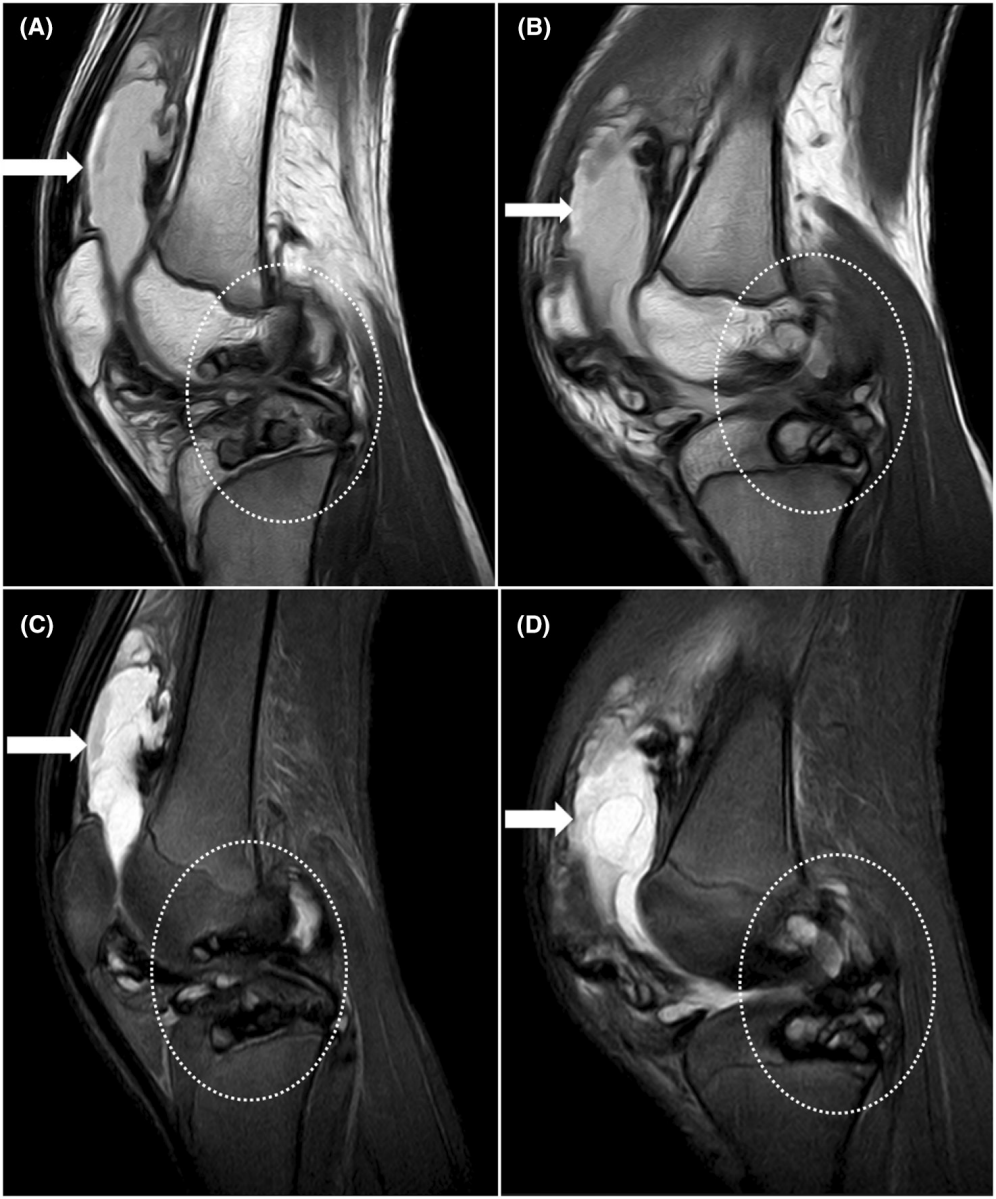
T1W (A, B) and PD (C, D) sagittal MRI images showing suprapatellar T1/PD hyperintense effusion (block white arrows) in patellofemoral compartment extending to suprapatellar recess suggesting hemorrhagic effusion. T1 and PD heterogeneously hyperintense lesions (dotted white arrows) in tibial plateau and medial femoral epicondyle suggesting hemorrhagic contents‐hemophilic pseudotumor.
